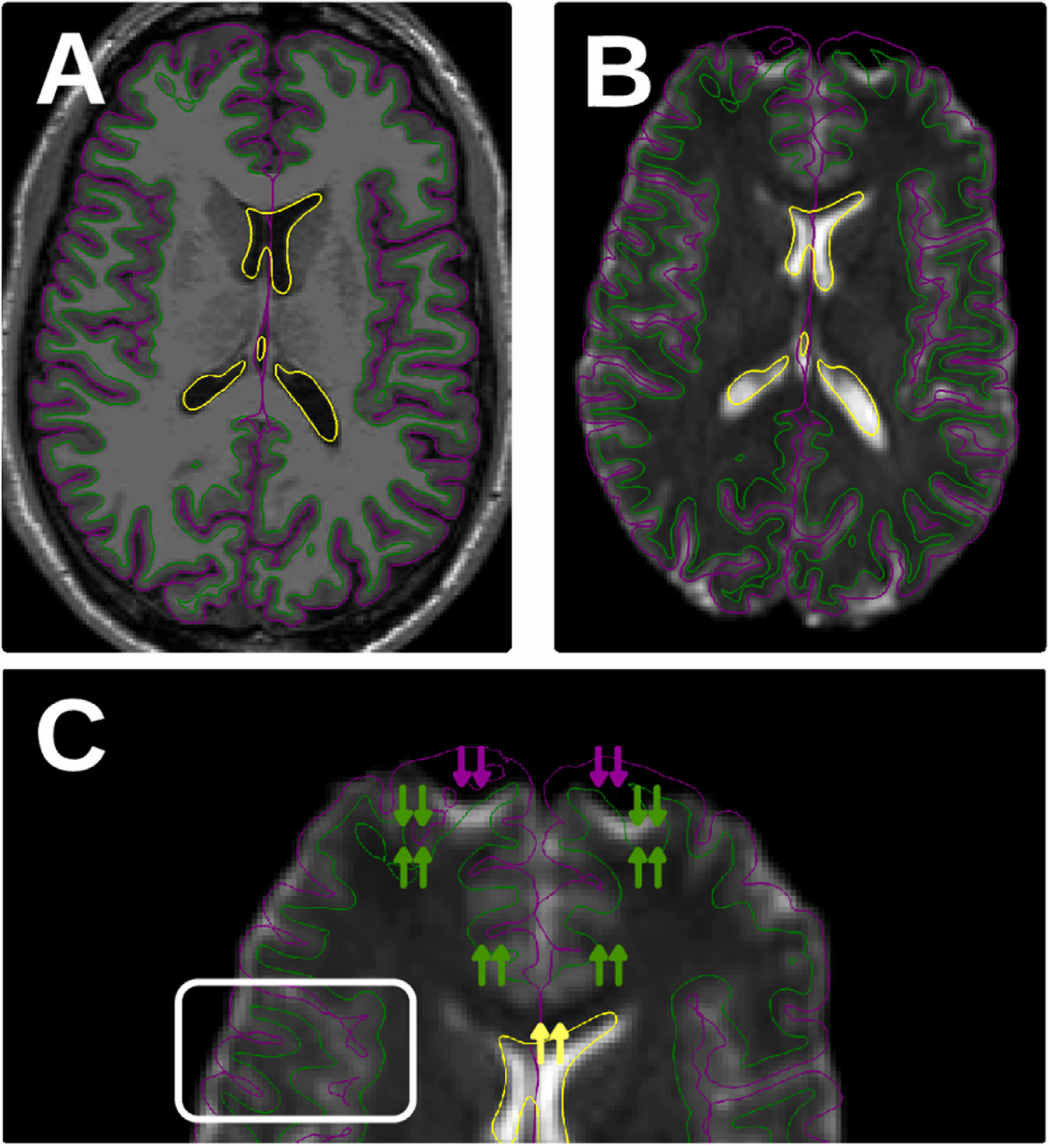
Susceptibility distortions are challenging in dMRI. The artifact causes a misalignment of the structures of the brain (represented by contours overlaid on the T1-weighted -T1w- image of panel A) and the dMRI data (as depicted in panel B). In panel C we present a close-up of the frontal lobe of the diffusion image, where the warping of the echo-planar image (EPI) produces a mismatch with respect the “anatomically-correct” surfaces extracted from the T1w image. The warping is aligned with the phase-encoding (PE) direction of the image. In this case (panels B, C) the PE direction is the anterior-posterior axis. Since the distortion is related to the inhomogeneity of the field inside the scanner, some regions are not excessively affected by the artifact (white box in panel C). In this data paper, the methodology and instruments to generate “*a priori*” known distortions from real subjects that can be used as “*golden*”-standard in the validation of registration and segmentation processing tools for diffusion MRI.
